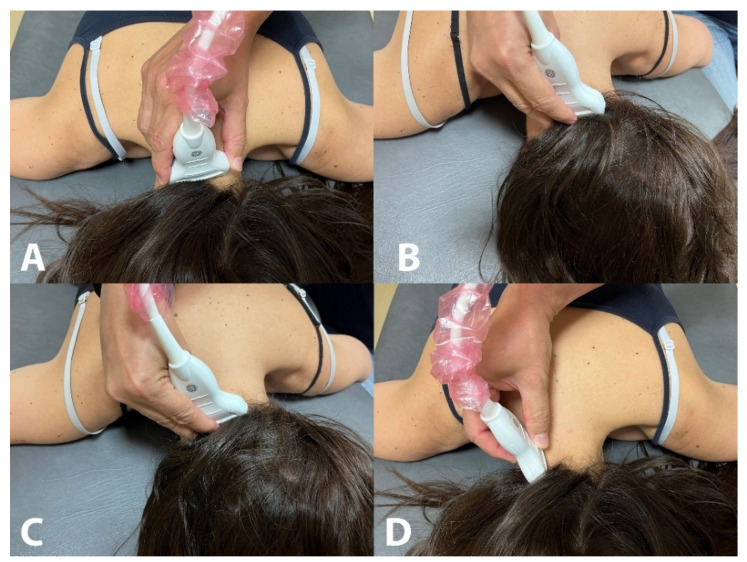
Placement of the ultrasound probe for the assessment of the rectus capitis posterior minor (A), rectus capitis posterior major (B), oblique capitis superior (C) and oblique capitis inferior (D).
